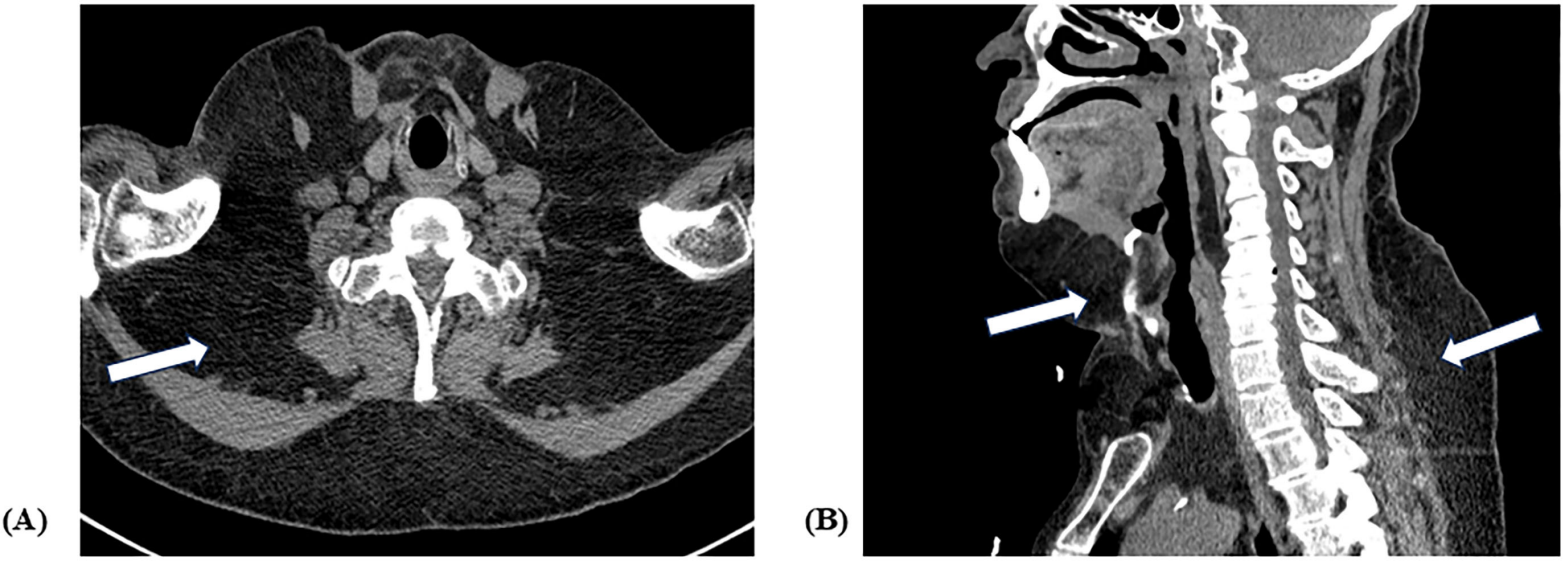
Axial (A) and coronal (B) CT images of the neck demonstrating diffuse fat accumulation (white arrows) in the anterior and posterior subcutaneous tissues, extending into the thoracic inlet.
